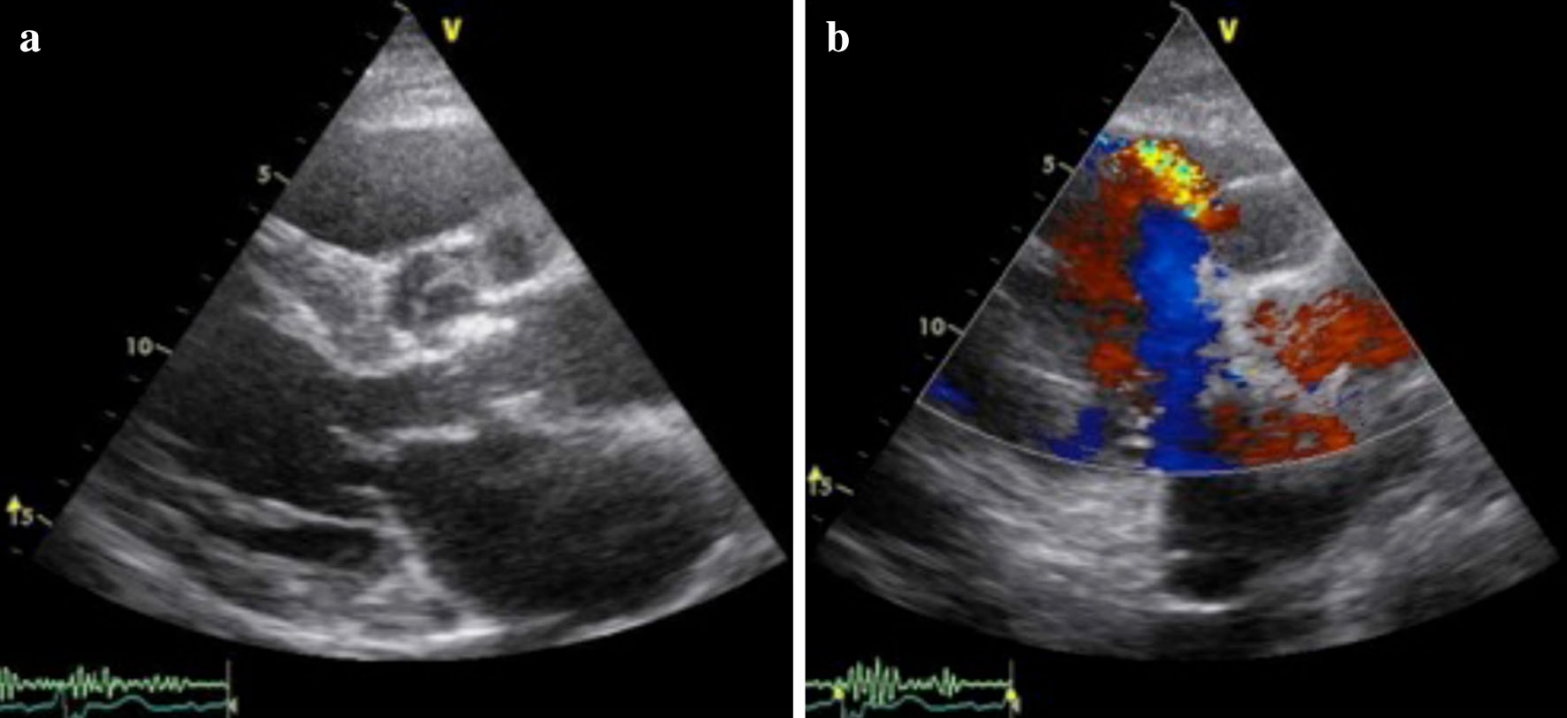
a Transthoracic echocardiogram showing vegetation on the bioprosthesis and annular abscess; b transthoracic echocardiogram showing shunt flow from the left to the right ventricle
